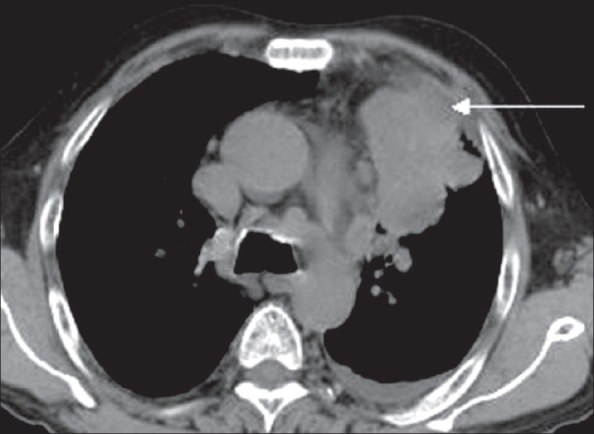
Plain CT scan shows a peripherally located left upper lobe mass with heterogeneous density, demonstrating a large area abutting the pleura (arrow), which is typically the preferred site for a biopsy
